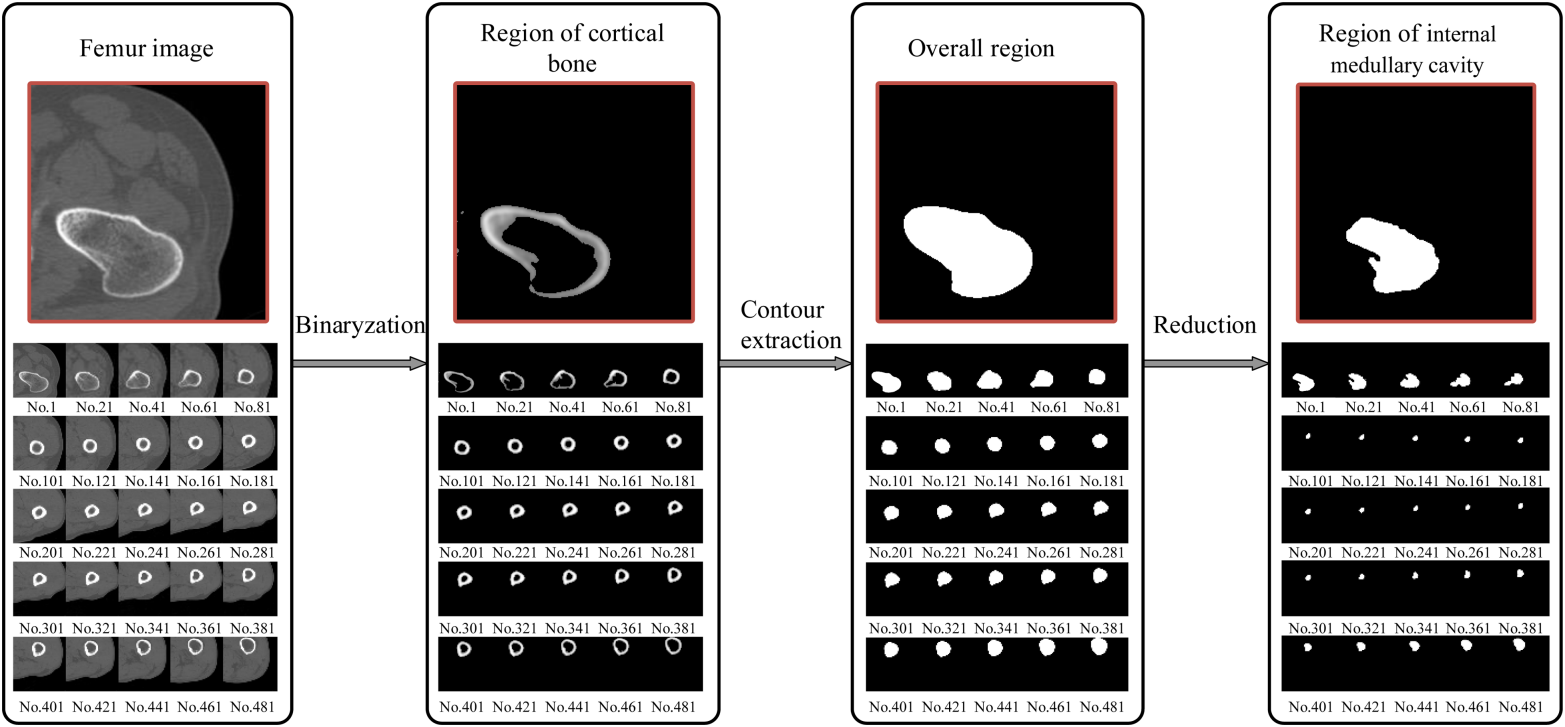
Extraction of the femur region of interest (ROI); from the proximal end to the distal end, one of every 20 CT images is selected. The final window size is 7 × 7, with a standard deviation (*σ*) of 3 and a threshold (*T*_0_) of 117. The size of the internal medullary cavity area, referred to as *s*_m_, describe the medullary cavity area in the transverse section. Similarly, the cortical area, eferred to as *s*_c_, describe the size of the cortical region in the transverse section.
